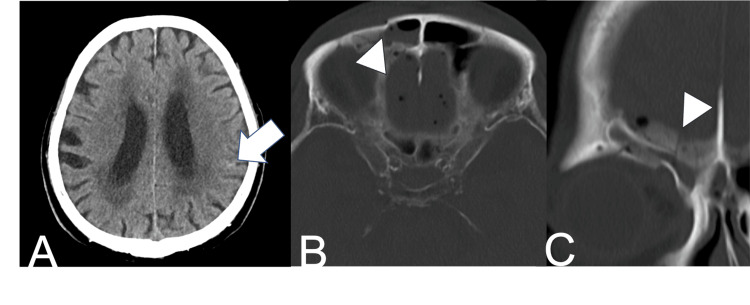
(A) Axial head CT scan at admission show mild traumatic subarachnoid hemorrhage (white arrow); Axial (B) and coronal (C) head CT scans at admission show anterior skull base fracture (arrowheads) and pneumocephalus.
